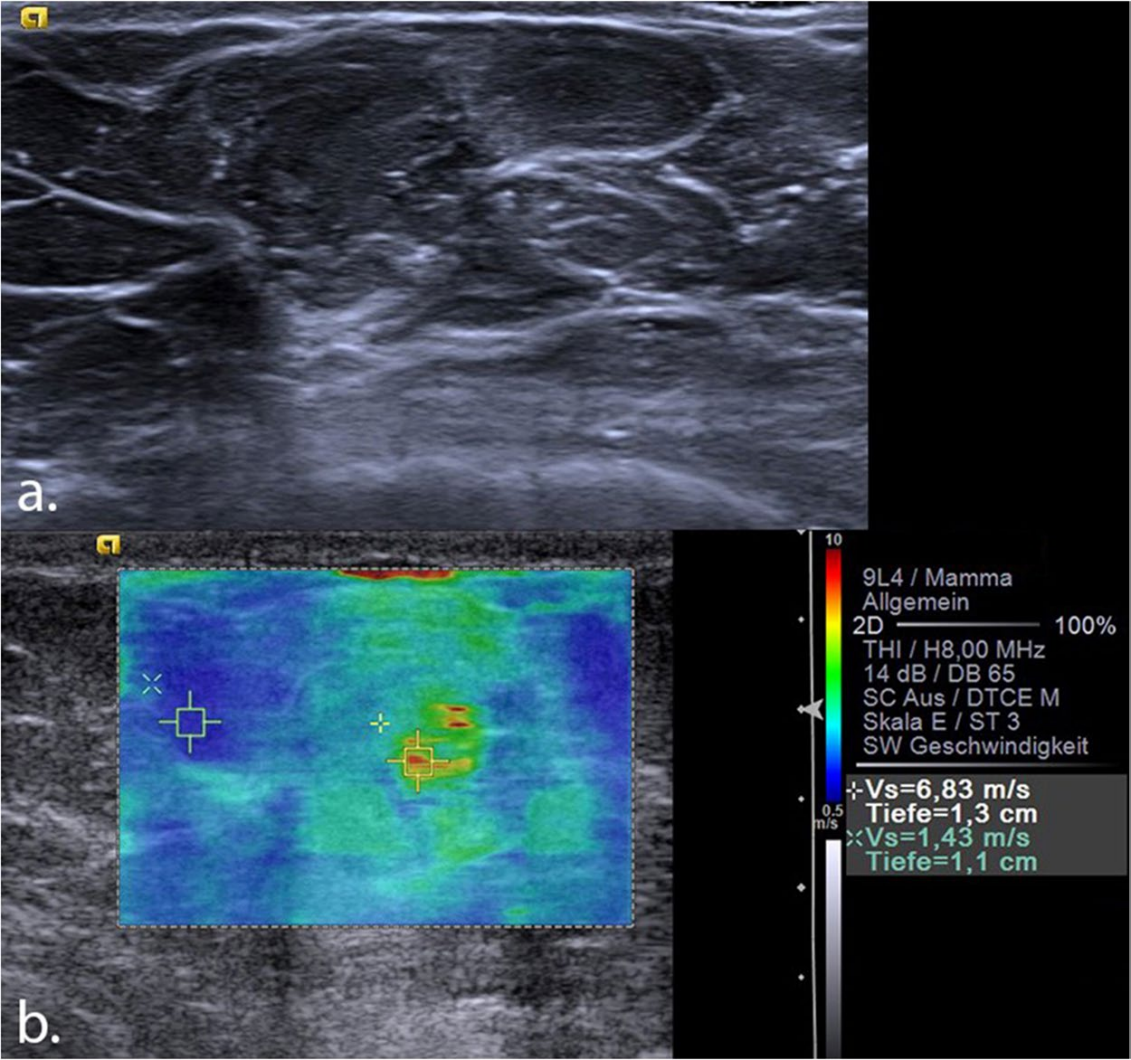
(a) Mucinous carcinoma seen in B-mode ultrasound as a 14 mm isoechoic, partially lobulated lesion with posterior enhancement, which was classified as BI-RADS 4b. (b) VTIQ elastography shows an overall hard lesion (scale adjusted to 10 m/s) with a maximum shear wave velocity of 6.83 m/s (above the “rule-in” threshold), indicating a BI-RADS 5 tumour. A further ROI measures the velocity inside fatty tissue (1.43 m/s). VTIQ: Virtual Touch IQ, ROI: region of interest.
